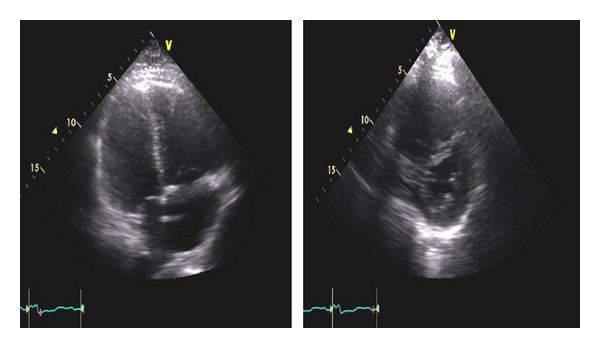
Systolic apical 4-chamber view with normal-sized left ventricle on the left and markedly dilated right ventricle on the right, (b) Parasternal short-axis view of left ventricle shows “D-shaped” left ventricle indicating right ventricular pressure overload and markedly dilated and thin-walled right ventricle on the top.
